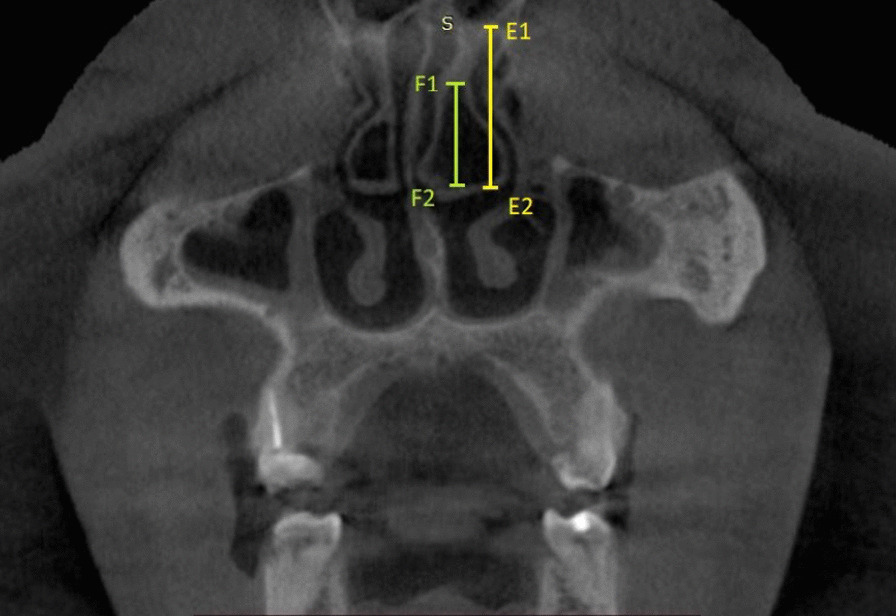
Coronal CBCT view showing the method used for identifying CB as per the criteria by Stallman et al. Line E1E2 represents the vertical length of the middle turbinate. Line F1F2 represents the extent of pneumatization caused by CB
